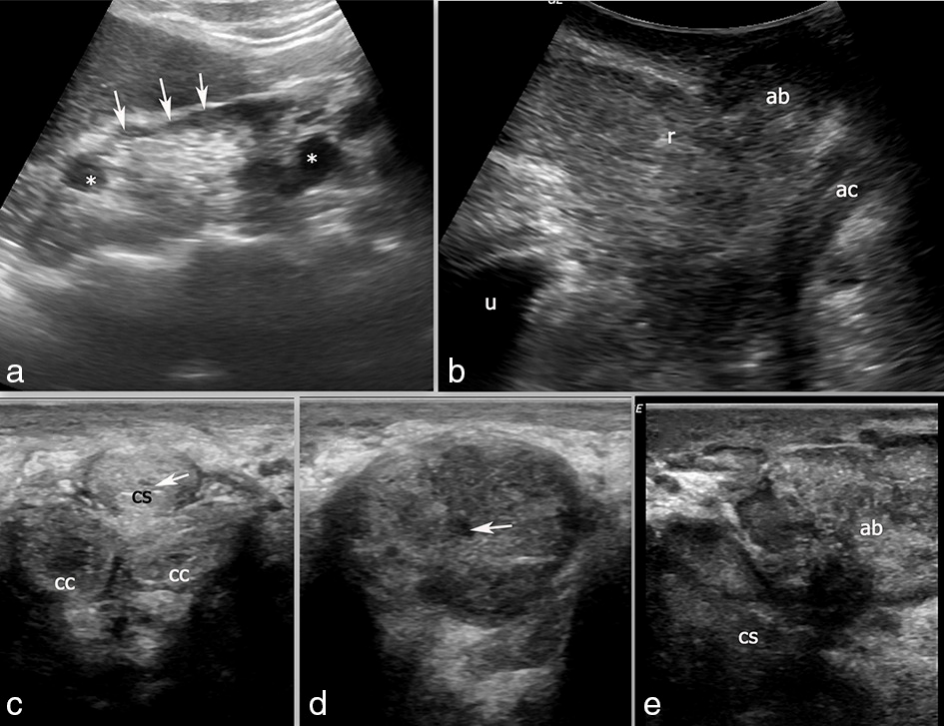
Pre-operative ultrasound images of the patient. (a) Longitudinal image of the left kidney showing cortical scarring (arrows) and caliectasis (asterisks). (b) Sagittal transperineal image shows an abscess (ab) in the perineum in relation to oedematous root of penis (r); the anal canal (ac) and urinary bladder (u) appear normal. (c) Axial image showing a normal proximal penile urethra (arrow) and normal parts of the corpus spongiosum (cs) and corpora cavernosa (cc). (d) Axial image showing a bulbar urethra (arrow) with an oedematous corpus spongiosum. (e) Sagittal transperineal image showing perineal abscess (ab) extending to the proximal corpus spongiosum (cs).
